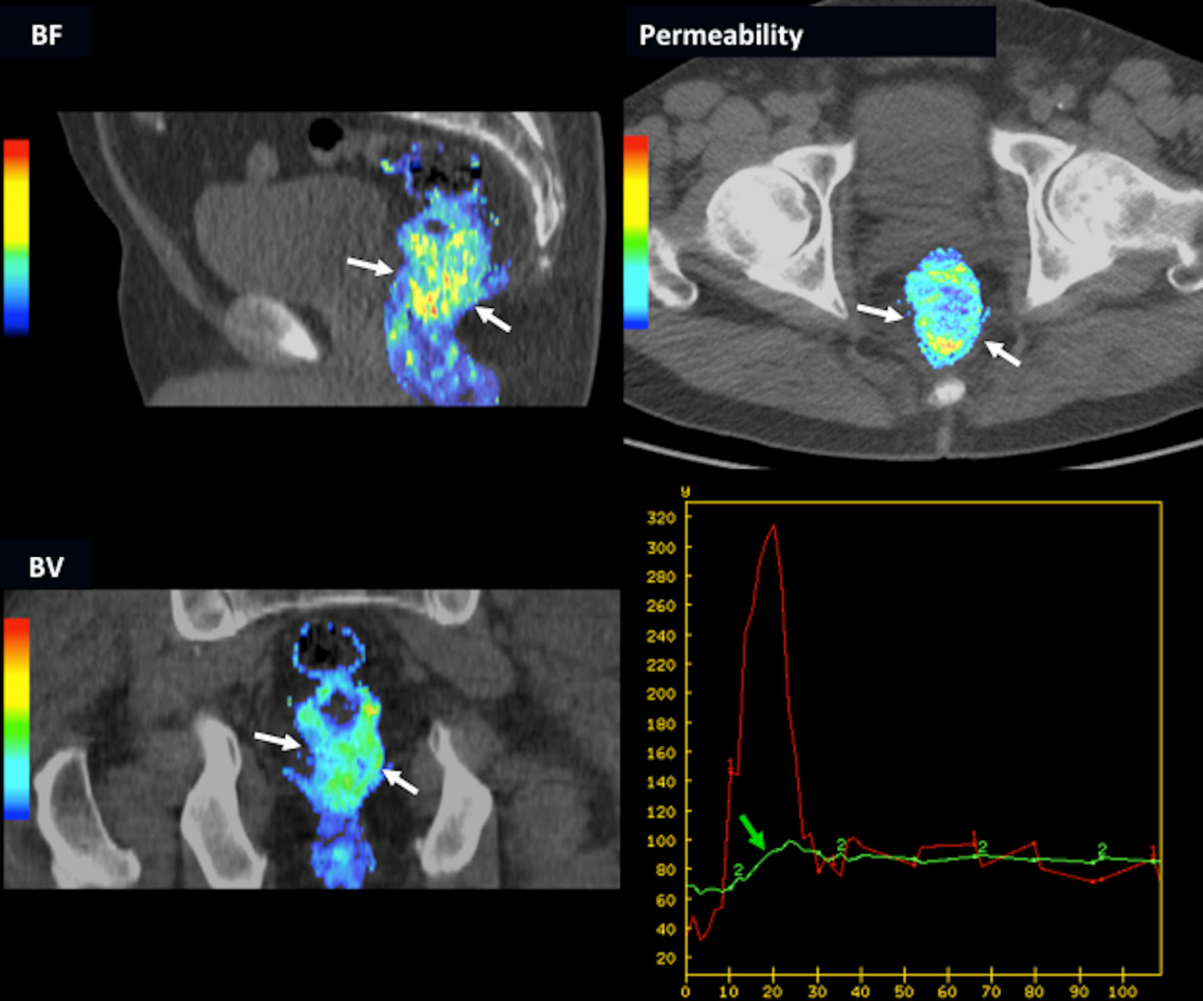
Volumetric perfusion CT multiplanar and multiparametric evaluation in a 62-year-old man with rectal cancer (white arrows). Parametric maps fused (50 % transparency) with CT images in different planes demonstrated increased values of perfusion-related parameters within the tumour: sagittal reformatted blood flow (BF) map, axial reformatted permeability map, and coronal reformatted blood volume (BV) map. Time-density curve of the tumour (bottom-right) demonstrated an enhancement curve type 3 (green arrow)
